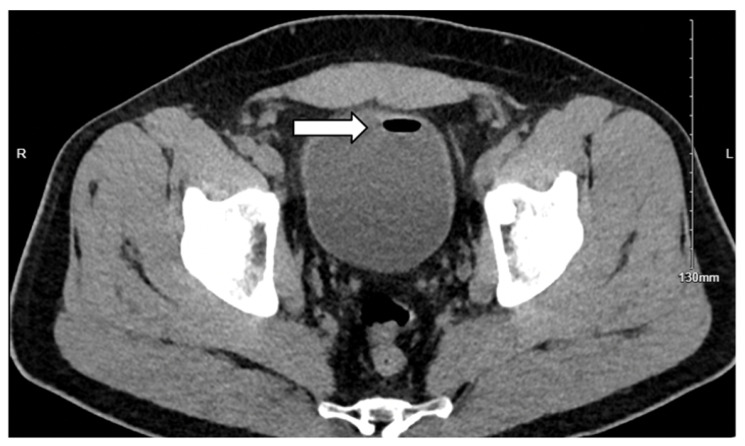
Axial noncontrast computed tomography image demonstrating the presence of air within the bladder (white arrow).
